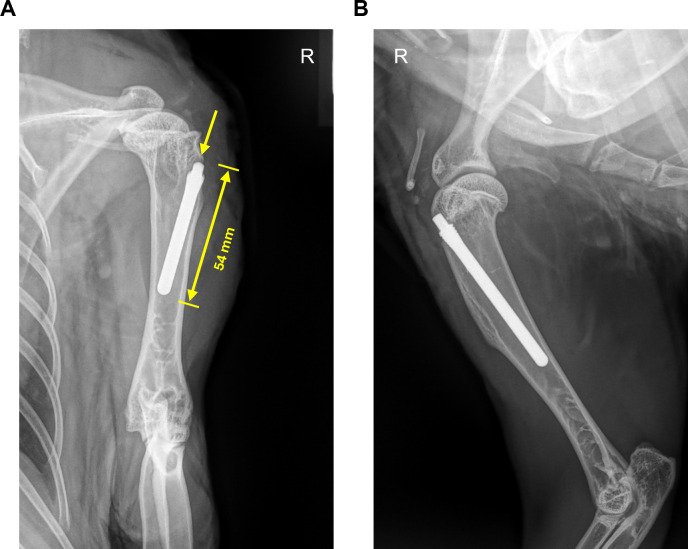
Radiographs of the right humerus taken immediately postoperatively. Craniocaudal (A), with the length of the intramedullary nail, and the insertion point indicated with the yellow arrow, and mediolateral (B).
